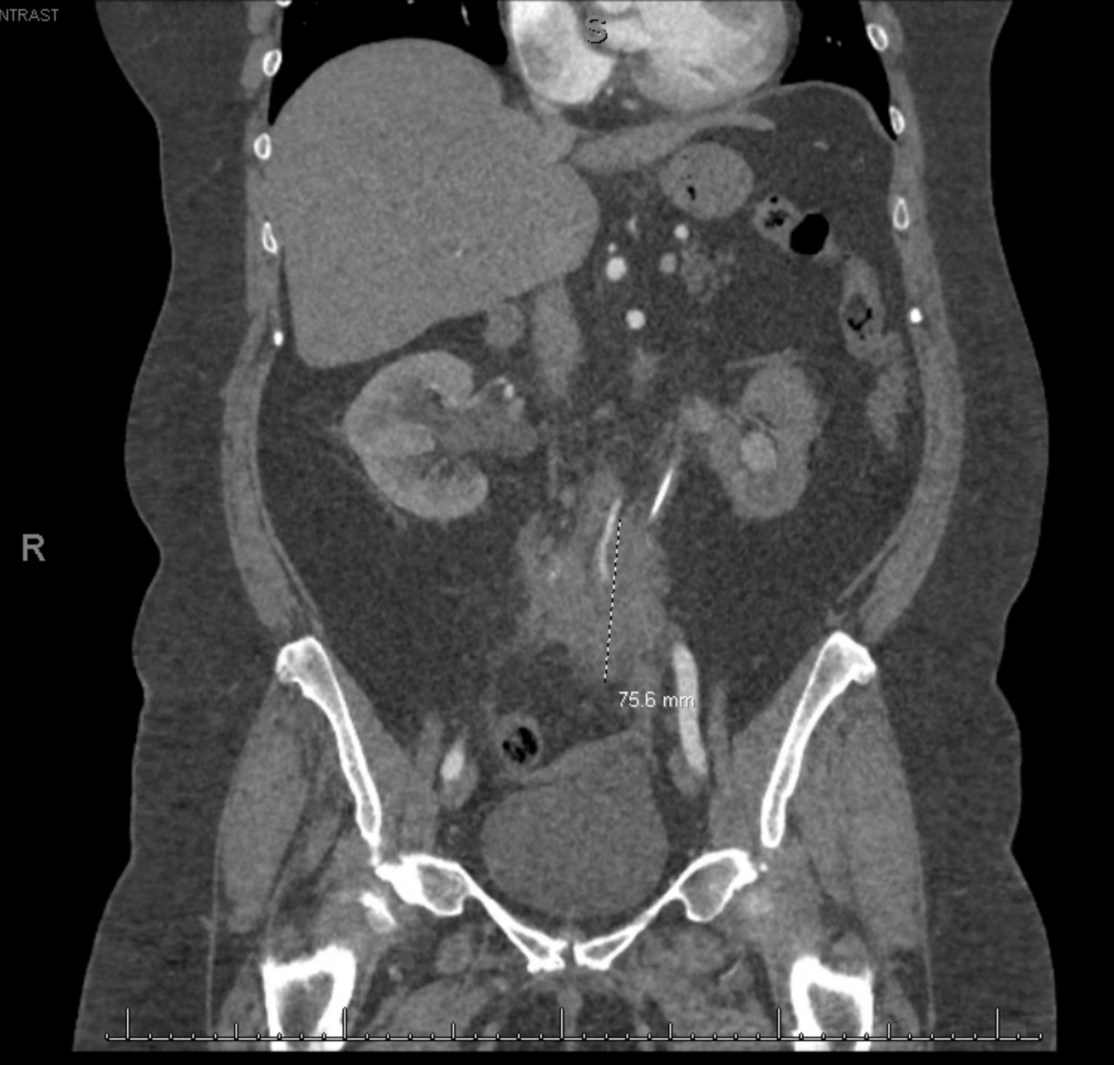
Subsequent CT scan, four months later, noting enlargement of the mass, now measuring 7.6 x 3.8 x 5.6 cm and encasing the right ureter causing mild right hydronephrosis.
